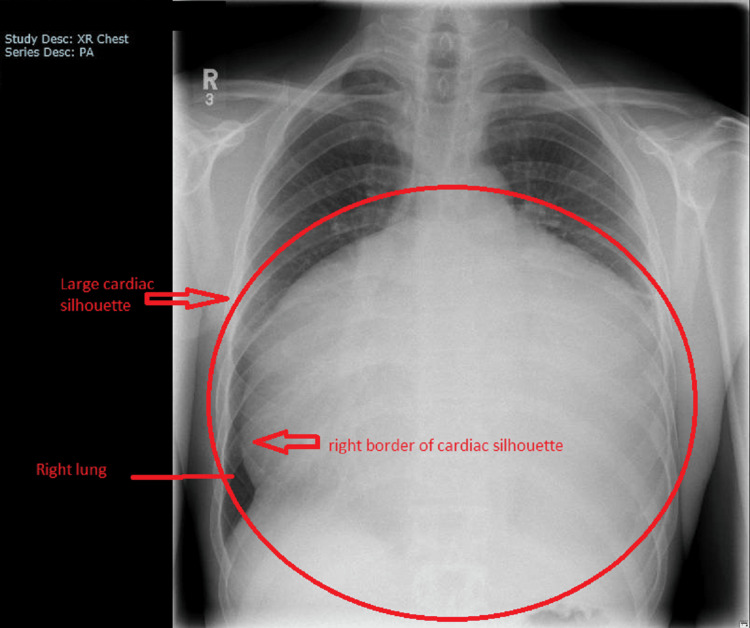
Chest X-ray Chest X-ray showing large cardiac silhouette suggestive of massive pericardial effusion
